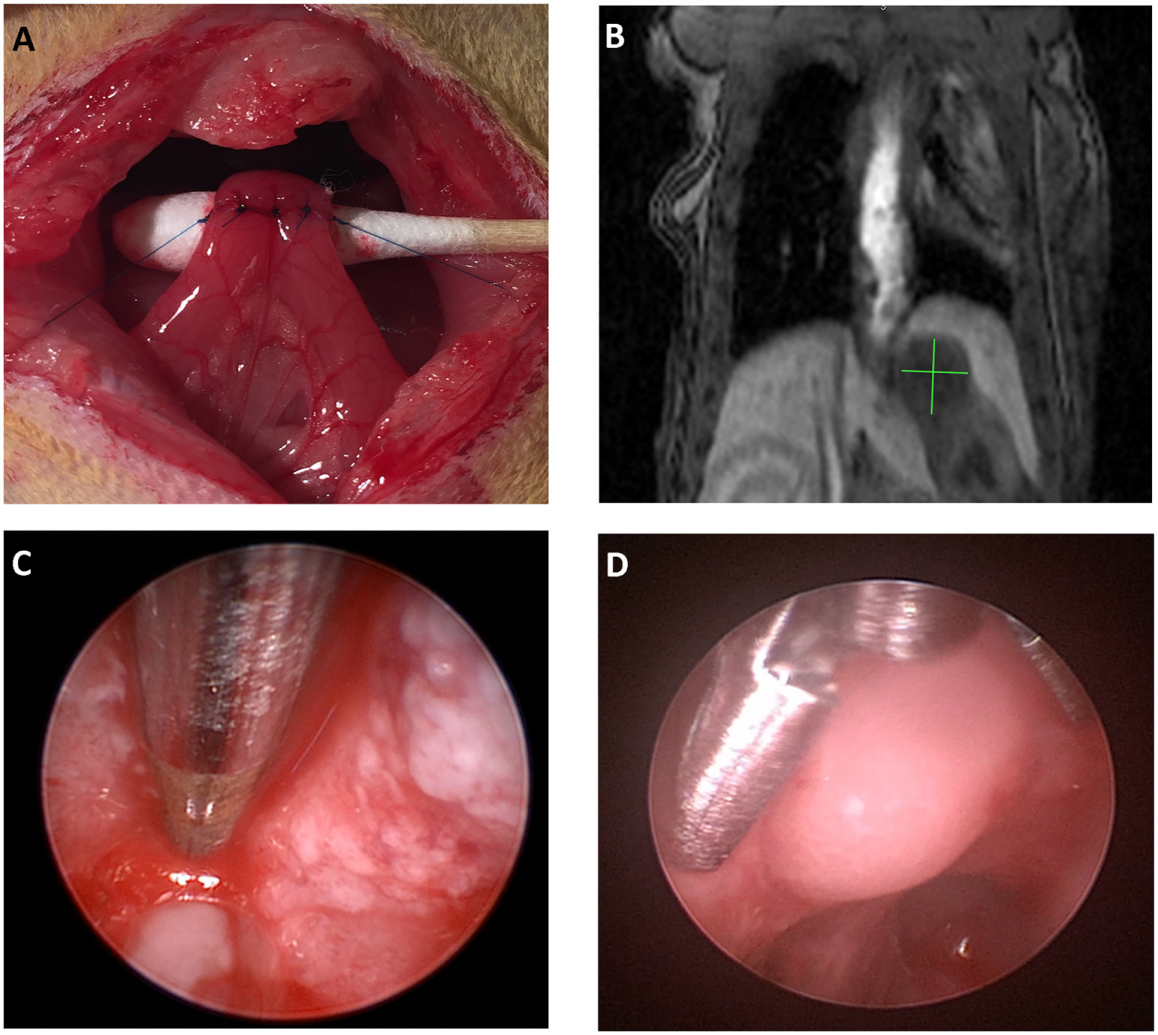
The modified levrat model. (A) End-to-side anastomosis of the esophagus with the jejunum performed as part of the modified Levrat surgery. (B) Coronal MRI image with a visible tumor at the esophagojejunal anastomosis. (C and D) Visual representation of an intratumoral injection and endoscopic biopsy of a suspected tumor in the modified Levrat model, respectively.
